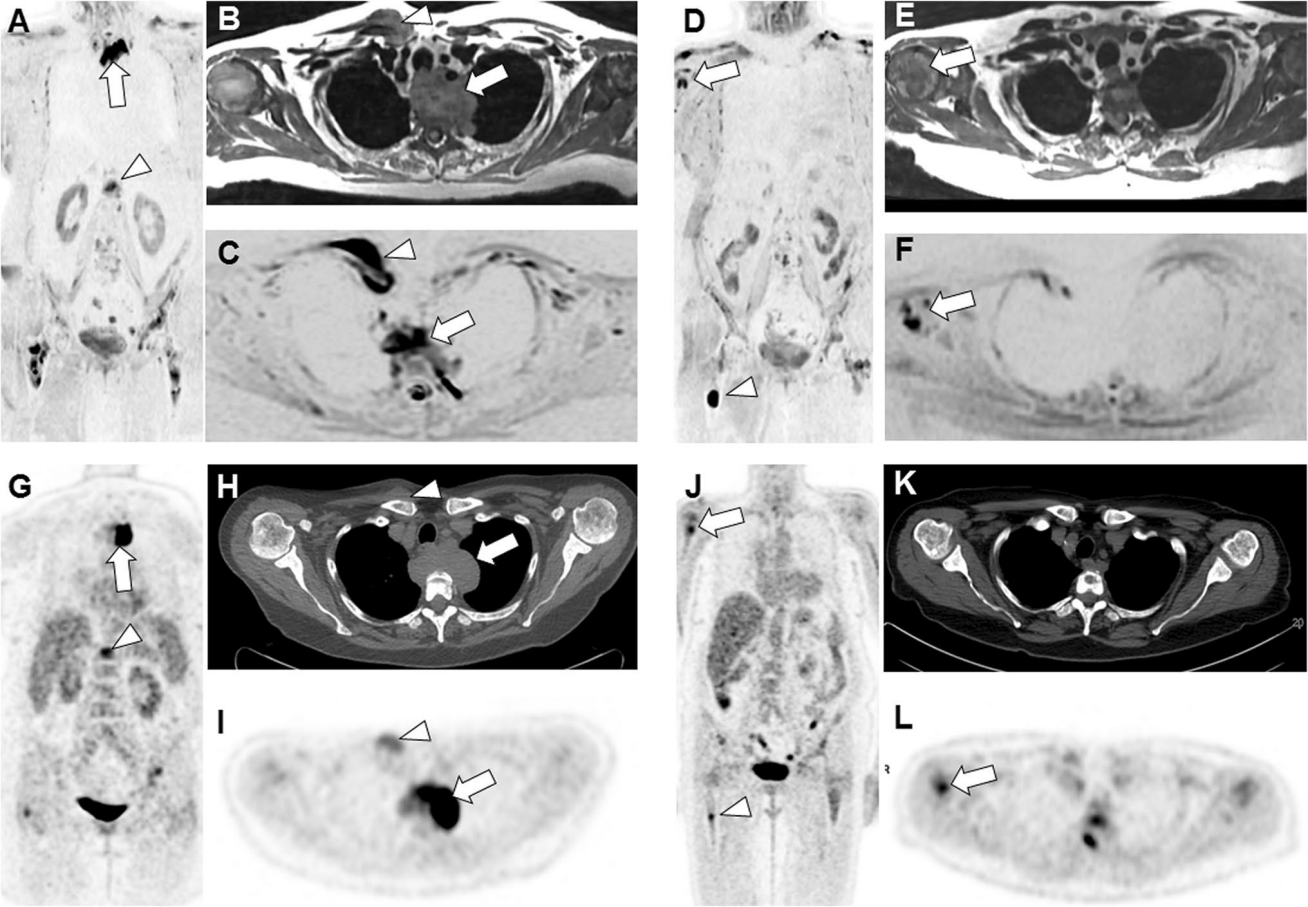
74 year-old woman with MM (IgG lambda stage IIIA, ISS 1 MM with adverse cytogenetics (del 17p), fourth line (salvage) therapy with Elotuzumab, Lenalidomide, and Dexamethasone): WB-MRI and PET/CT performed for evaluation of disease and treatment response after 3 cycles, biological progression. WB-MRI and PET show bone and extramedullary disease, heterogeneity of response and residual disease. A-C Baseline MRI findings. Coronal DWI MR image (B = 1000 s/mm^2^) (A) shows active bone lesions within both femurs, within the T12 vertebra (arrowhead), and paramedullary mass in the superior and anterior mediastinum (arrow). Transverse T1-weighted (B) and DWI (C) MR images confirm the presence of para-medullary extension adjacent to upper thoracic vertebra (arrow) and to the medial portion of the right clavicle (arrowhead). D-F: Post-treatment MRI findings. Coronal DWI (D), and transverse T1 and DWI MR images show major response of almost all lesions, including the paramedullary extension in the mediastinum and right clavicular regions. The coronal image and the transverse images show new lesions within the right humerus (arrows in D-F) and right femur (arrowhead in D). G-I Corresponding PET/CT findings. Coronal PET image shows exactly the same findings: femoral, T12 (arrowhead) and mediastinal lesions (arrow)(SUVmax of femoral, T12 and mediastinal lesions:: 7.3, 16.9, 12.7; mediastinal blood pool: SUVmax 2.1). Corresponding transverse CT (H) and PET (I) images show soft tissue lesion adjacent to an upper thoracic vertebra (arrow) and to the medial part of the right clavicle (arrowhead). J–L Follow-up PET/CT findings. Corresponding PET/CT images show exactly the same findings: major response of almost all lesions including paramedullary extension in the mediastinum and right clavicular region, but appearance of new foci within right humerus (arrows in J and L) and femur (arrowhead in J) (SUVmax of femoral, T12 and mediastinal lesions: 4.3, 4.6, 4.3; new humeral lesion: SUVmax 5.6; new femoral lesion: SUVmax 5.3; mediastinal blood pool: SUVmax 2.3)
